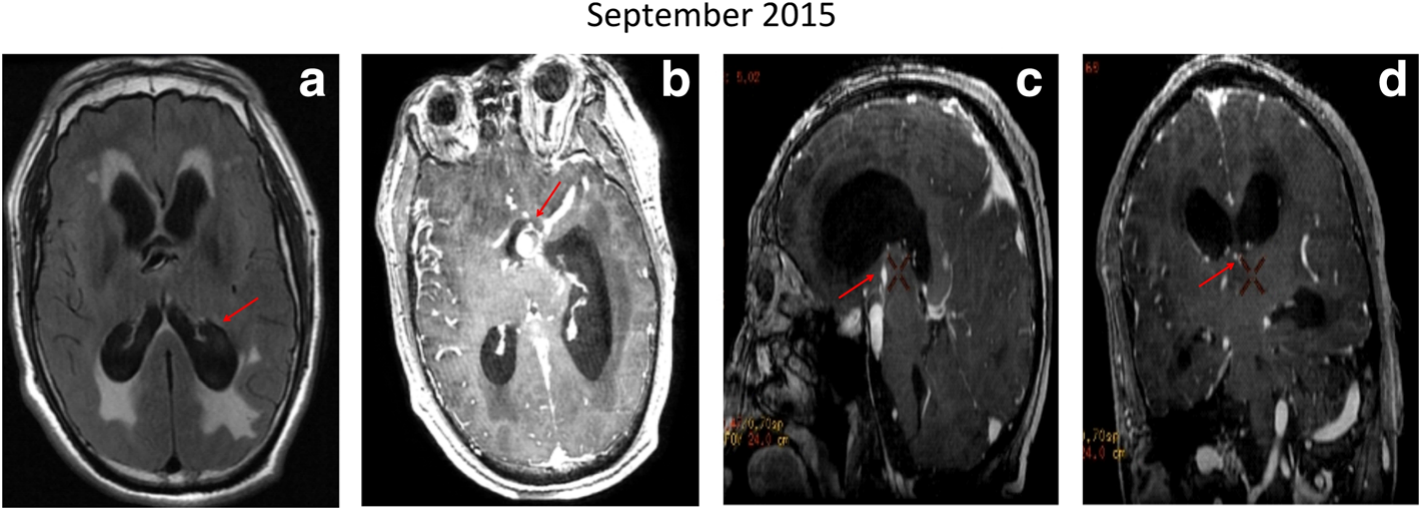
Non-enhanced and contrast-enhanced MRI scans. (a) Axial T1-weighted image showing a dilated lateral ventricle and encircled calyptriform with high intensity (red arrow). (b) Enhanced axial T1-weighted image showing BA vessel wall hematoma (hyperintensities and double lumen, red arrow). (c) Enhanced sagittal T1-weighted image showing bifurcation toward the base of the lateral ventricle (red arrow), a narrowed aqueduct of sylvius, a distorted third ventricle, and dilation of the lateral ventricle. (d) Enhanced coronal T1-weighted image revealing compression of the foramen of Monro due to VBD
